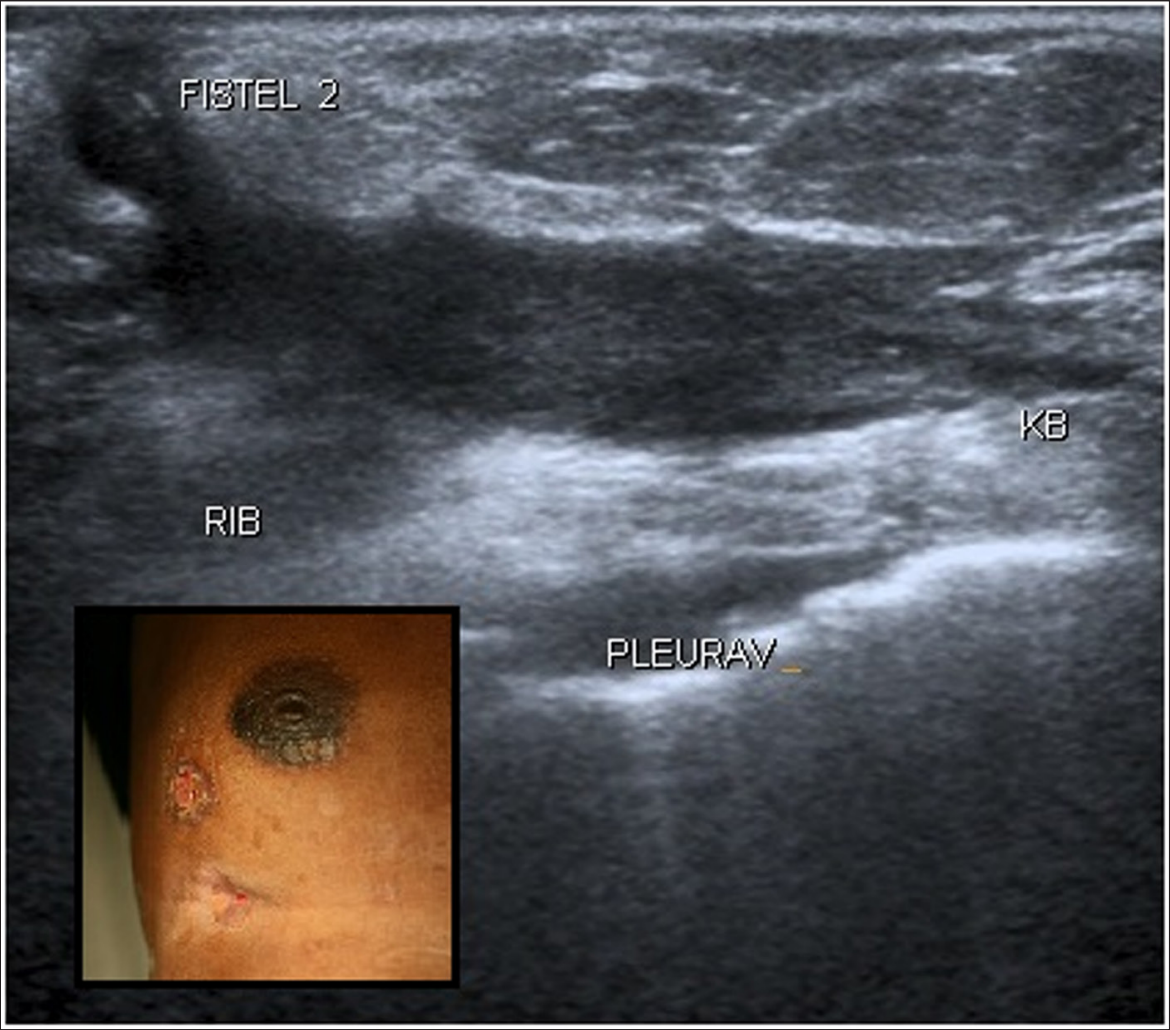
Ultrasound of the breast. Subfigure: Clinical presentation.
